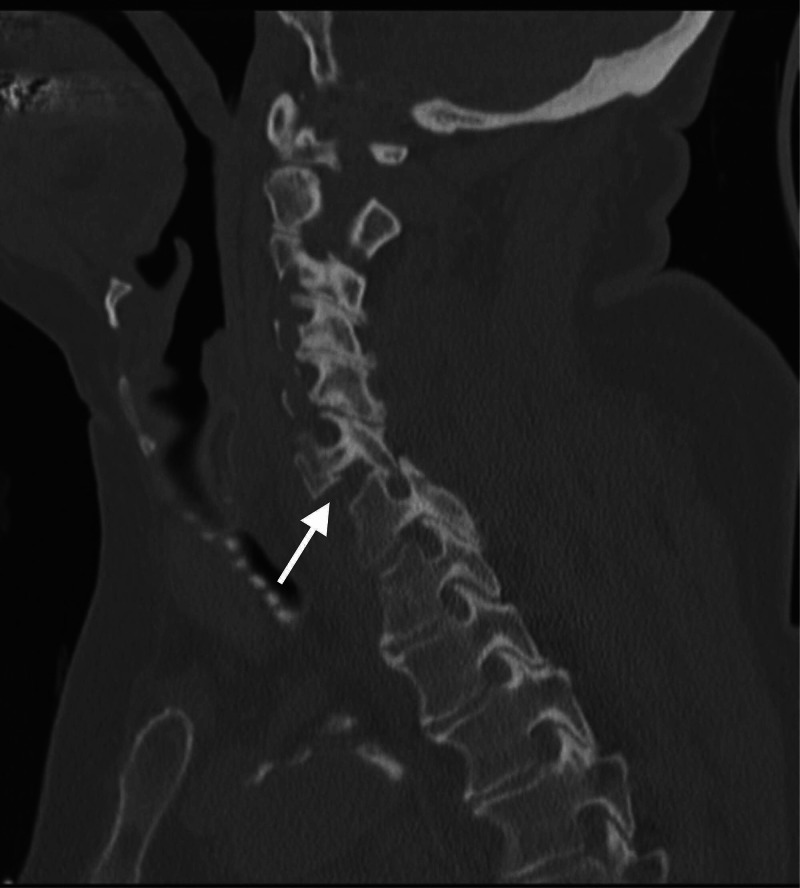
Sagittal cuts of the cervical spine CT scan demonstrating unilateral facet dislocation at the C6-C7 level Case courtesy of Associate Prof. Frank Gaillard, https://radiopaedia.org/, rID: 35609
